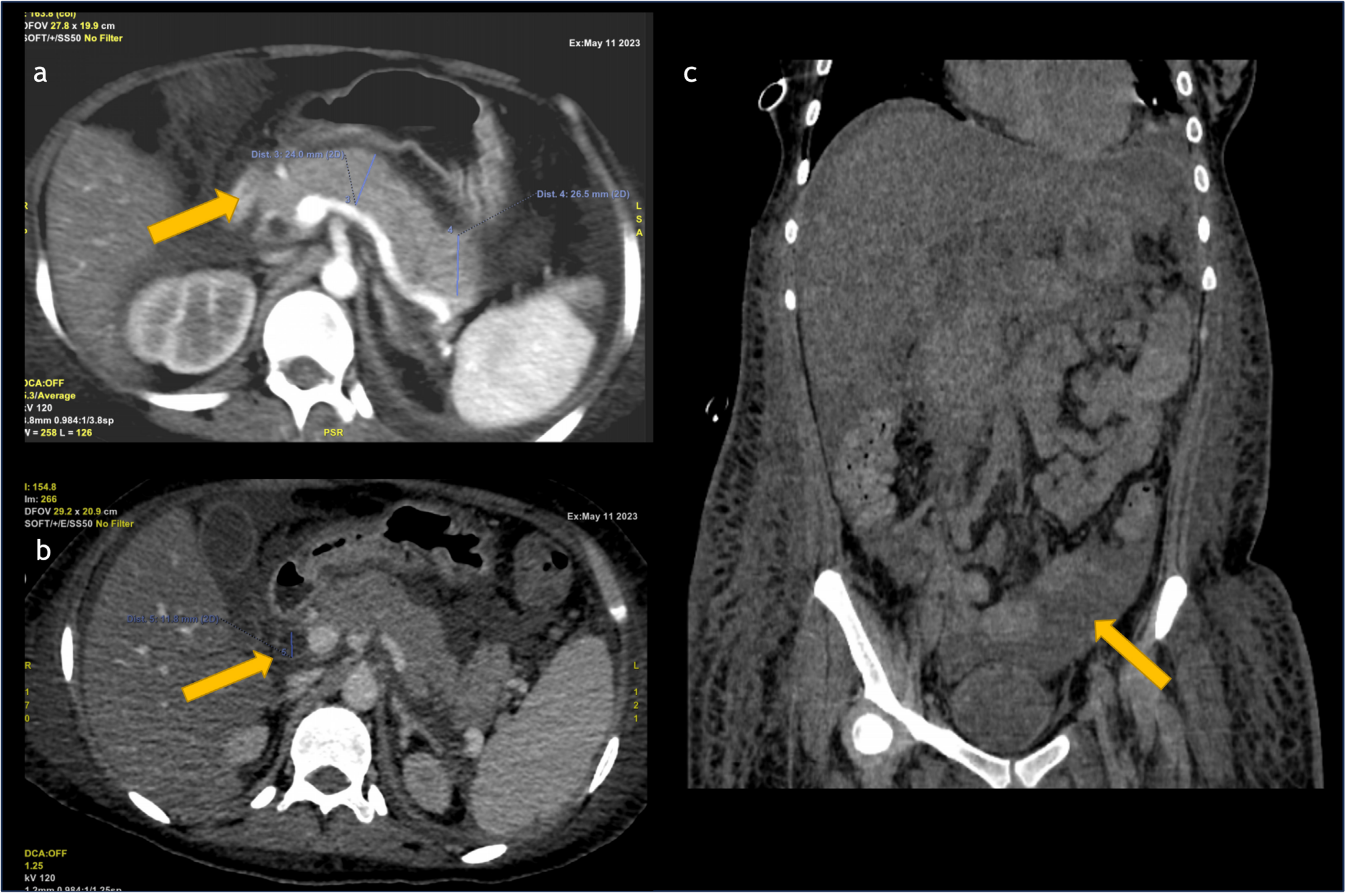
(a) Contrast‐enhanced computed tomography (CT) image of the pancreas showing significant pancreatic enlargement with a hypodense area measuring 24.0 mm (yellow arrow), suggestive of acute inflammation or necrosis. There is notable peripancreatic fat stranding and fluid collection, indicating acute pancreatitis with a potential developing pseudocyst. (b) Axial CT image of the abdomen demonstrating an enlarged pancreas with an inflamed area measuring 11.8 mm (yellow arrow). The image shows peripancreatic fluid accumulation and fat stranding, consistent with acute interstitial edematous pancreatitis. (c) Coronal CT image showing free fluid in the abdominal cavity (yellow arrow), commonly associated with acute pancreatitis.
